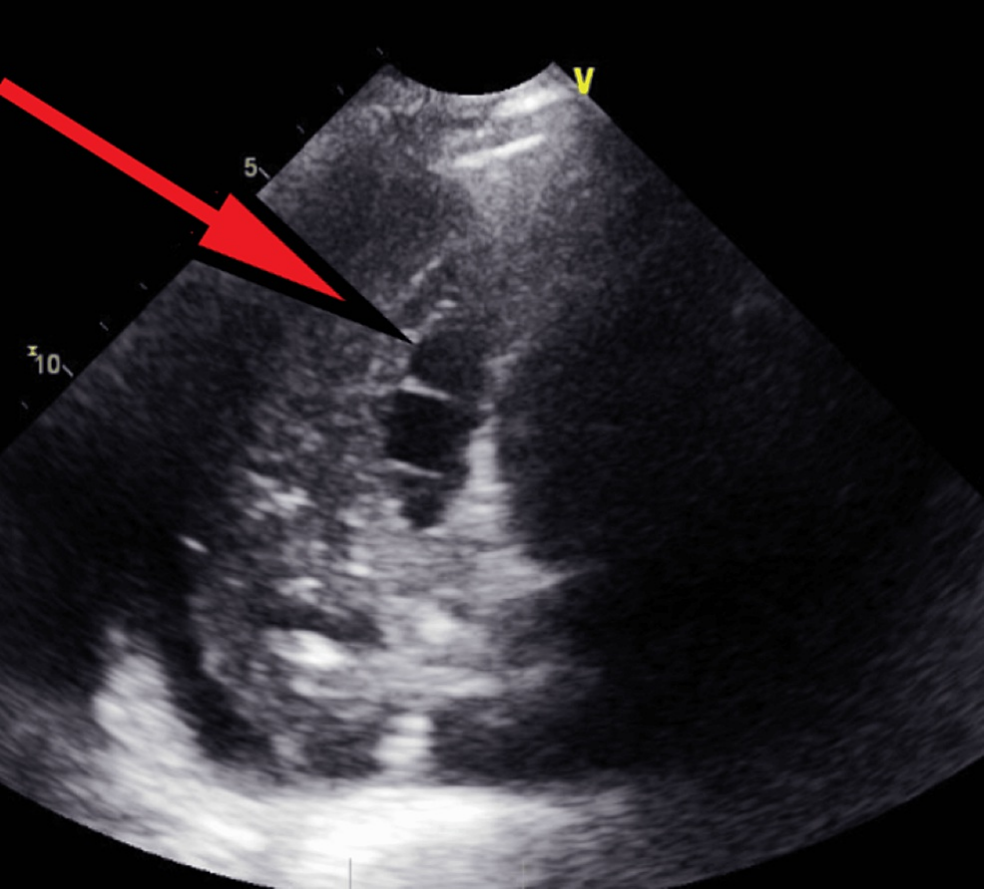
Transthoracic echocardiography post-pericardiocentesis - image 4 Transthoracic echocardiography post-pericardiocentesis; apical 4-chamber view showing another image of multiple loculations (red arrow) with some remnant pericardial fluid collections and the presence of septal bounce
